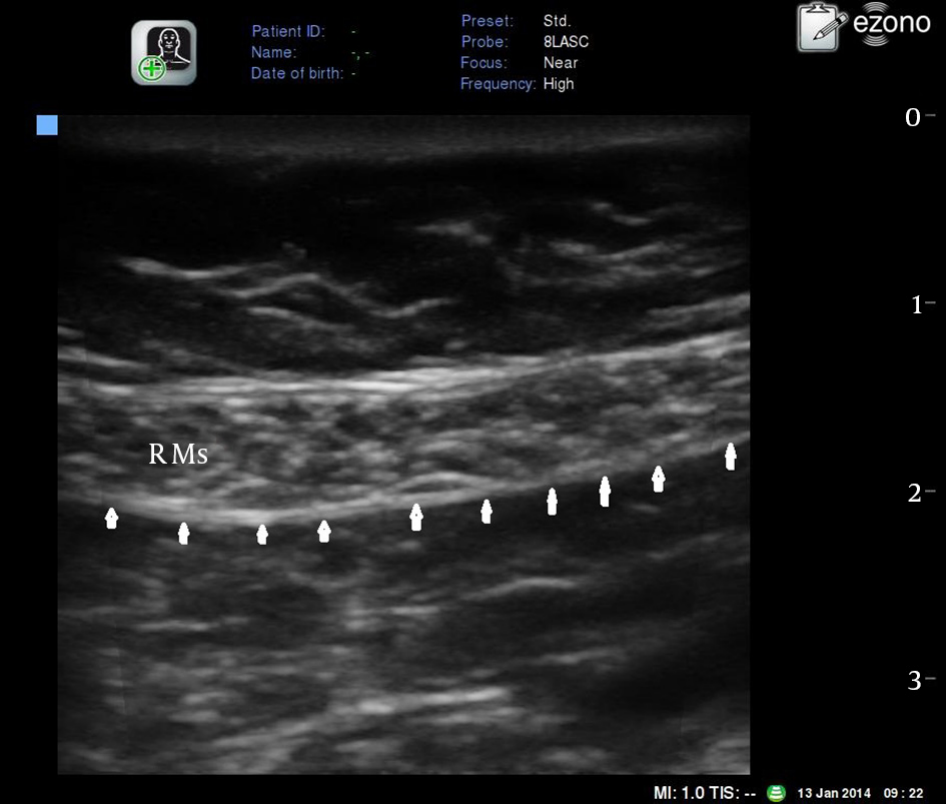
Transverse Ultrasound View of the Rectus Muscle Just Above the Umbilicus R Ms: rectus abdominis muscle. Arrows: posterior rectus sheath.
